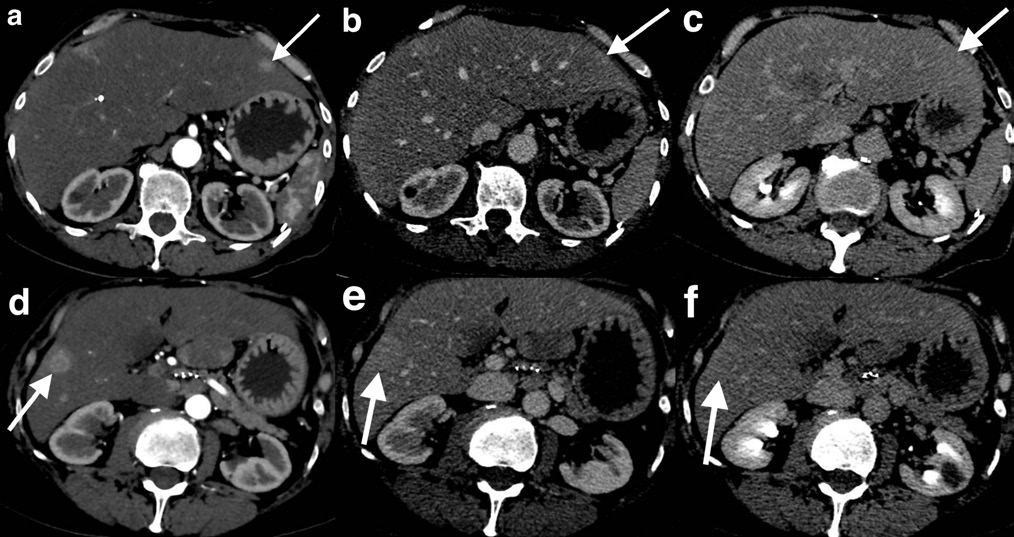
Hypervascular GIST liver metastases. Multiphase CT demonstrates a segment two lesion which diffusely enhances in the arterial phase (a). It is difficult to see in the portal venous phase and appears subtly hyperattenuating (b). It is isoatttenuating in the delayed phase (c). A second lesion at the junction of segments 5 and 6 exhibits rim enhancement in the arterial phase (d). In the portal venous phase, it shows mildly diffuse hyperattenuation with a wedge-shaped perfusion abnormality (e). In the delayed phase, the lesion is isoattenuating with a subtly enhancing rim (f). GIST, GIST, gastrointestinal stromal tumour/.
